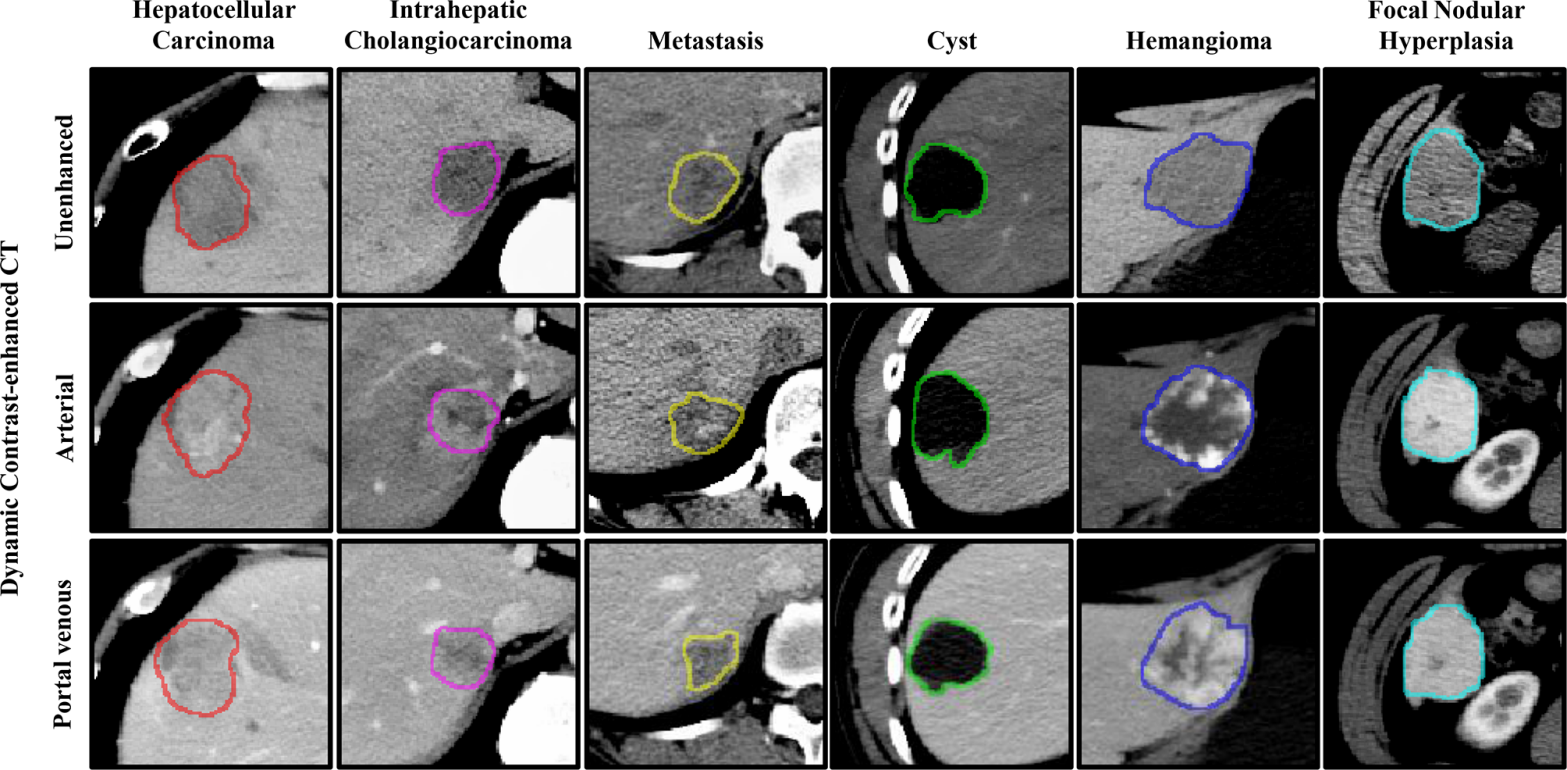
Representative images of focal liver lesions (FLLs) in different phases of dynamic contrast-enhanced computed tomography (CT). The contours represent the manually labeled boundary of the nodules.
